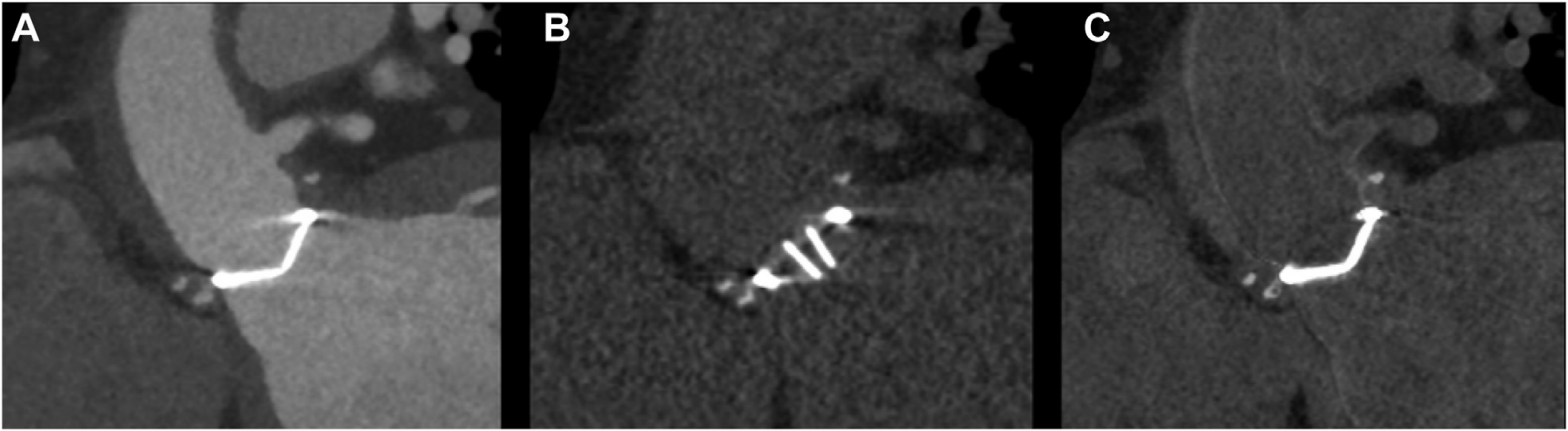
Pledgets used in surgical aortic valve replacement shown on computed tomography in contrast-enhanced, true non-contrast, and virtual non-contrast images. (A) Contrast-enhanced image, (B) true non-contrast image, (C) virtual non-contrast image.
